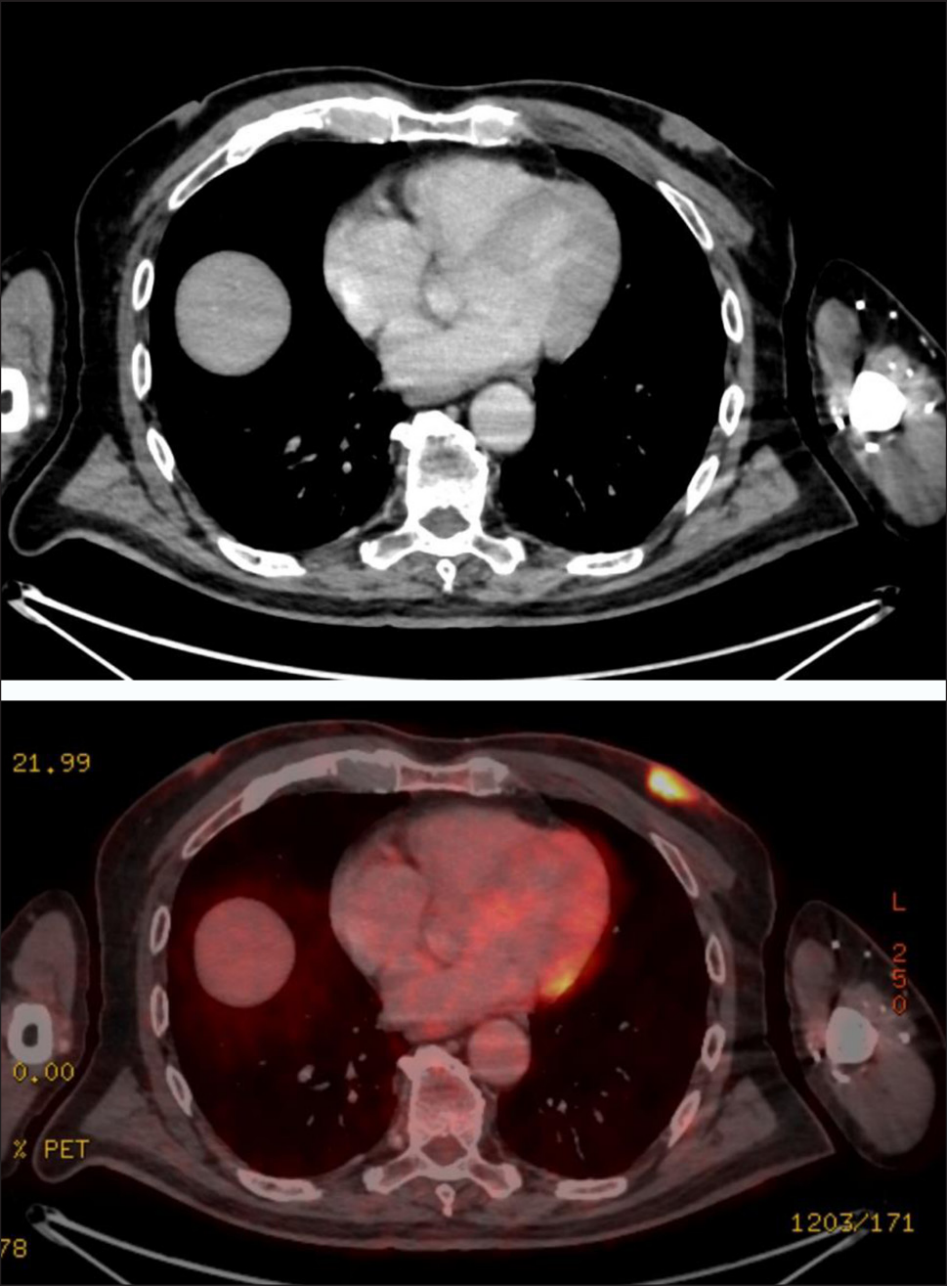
Metabolic active mass in the left breast on PET-CT.
